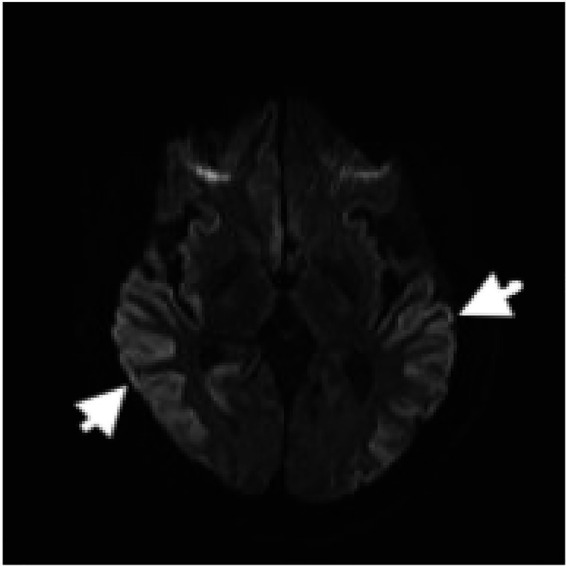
Axial DWI sequence showing non-asymmetric high signal in bilateral cortex (white arrows) of patients with Creutzfeldt-Jakob disease.
